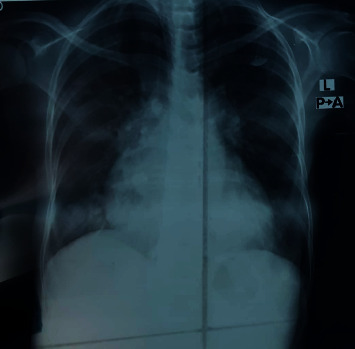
Chest X-ray posterior/anterior view revealed upper lobe diversion and congested hilar shadows and patchy consolidation in lower lobes (features of pulmonary edema and cardiomegaly).
